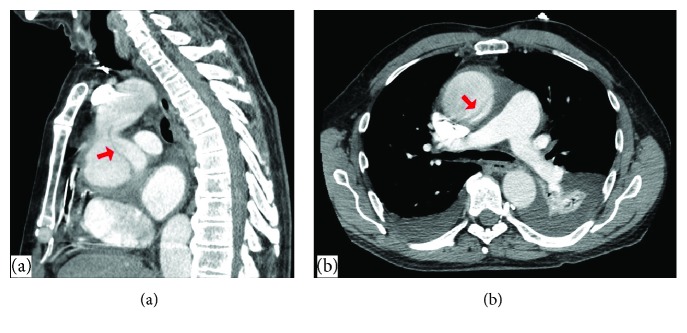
CT scan of the abdomen showing acute ascending thoracic aortic aneurysm measuring up to 7.5 cm with a dissection of the proximal ascending thoracic aorta originates approximately 1.3 cm above the aortic root. (a) Sagittal view of dissection site and (b) coronal view of dissection site. The area of dissection highlighted with an arrow.
